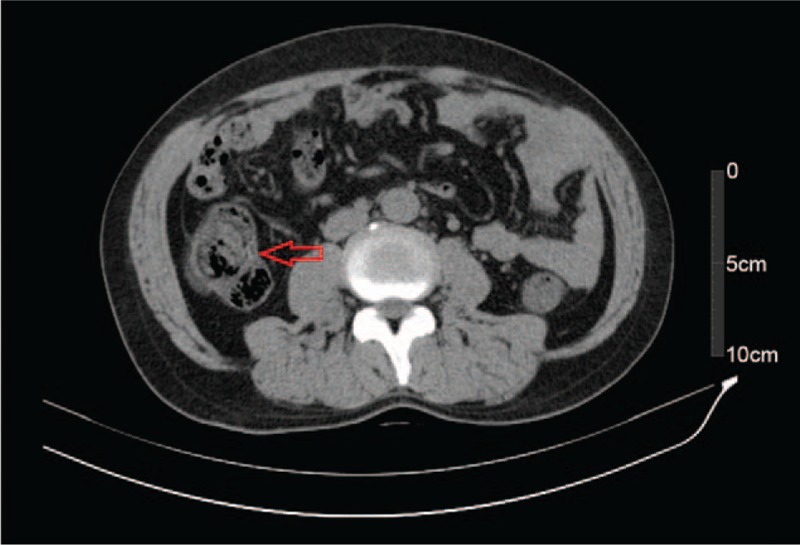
Abdominal computed tomography after admission showed eccentric thickening of the colon walls (red arrow).
